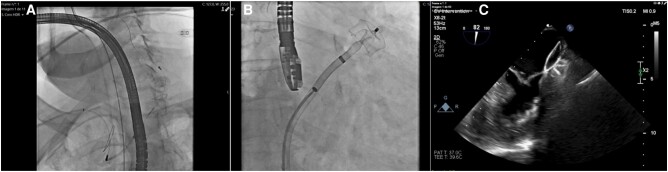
Percutaneous left atrial appendage occlusion. (*A*) SENTINEL™ (Boston Scientific, MA, USA) deployment. (*B*) Amplatzer Amulet™ (Abbott, IL, USA) deployment. (*C*) Transoesophageal echocardiography revealing good final result.
